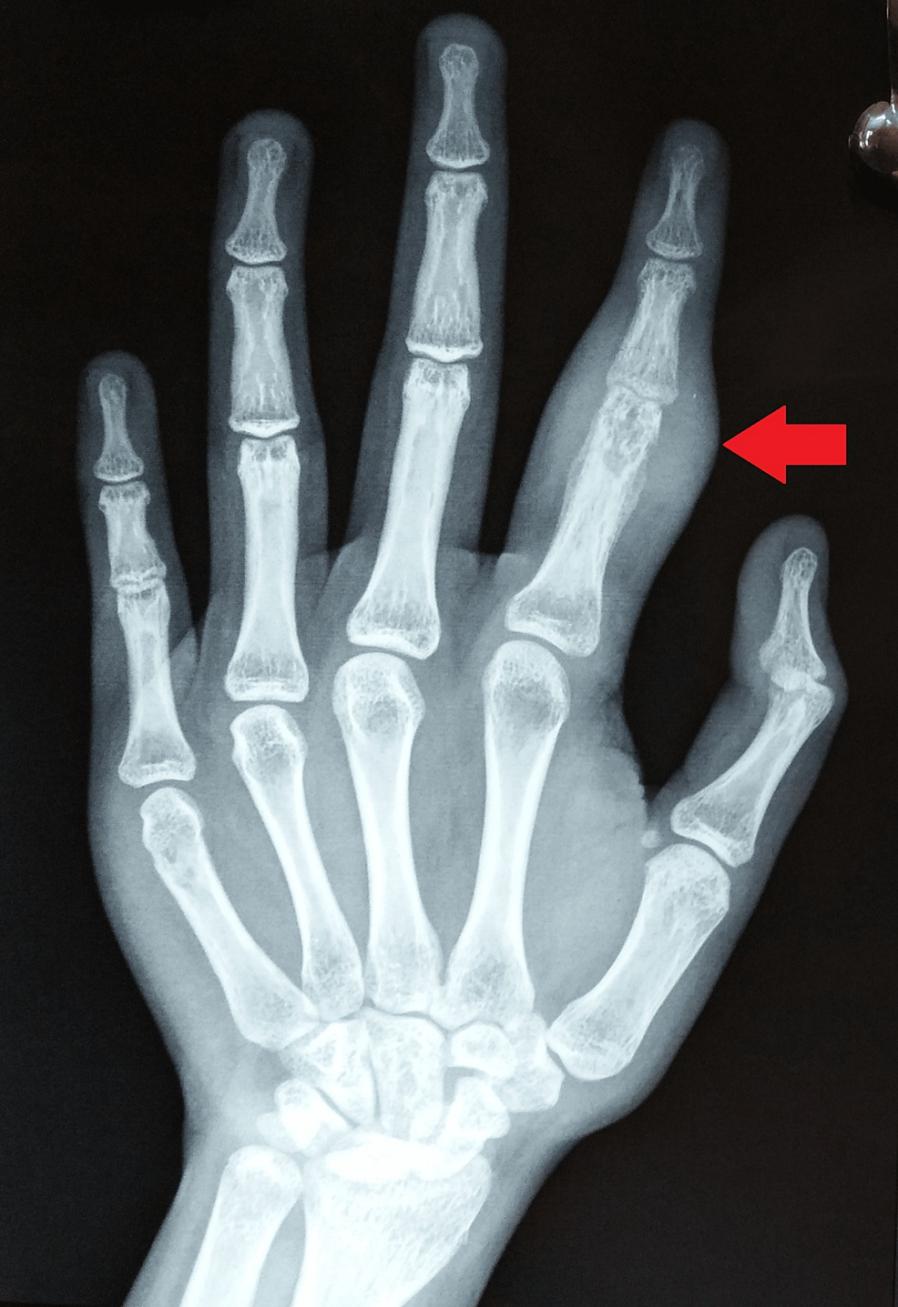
Plain radiographs of the left hand (postero-anterior) view showing soft tissue swelling with cortical erosion in the distal part of the proximal phalanx and a slight periosteal reaction
